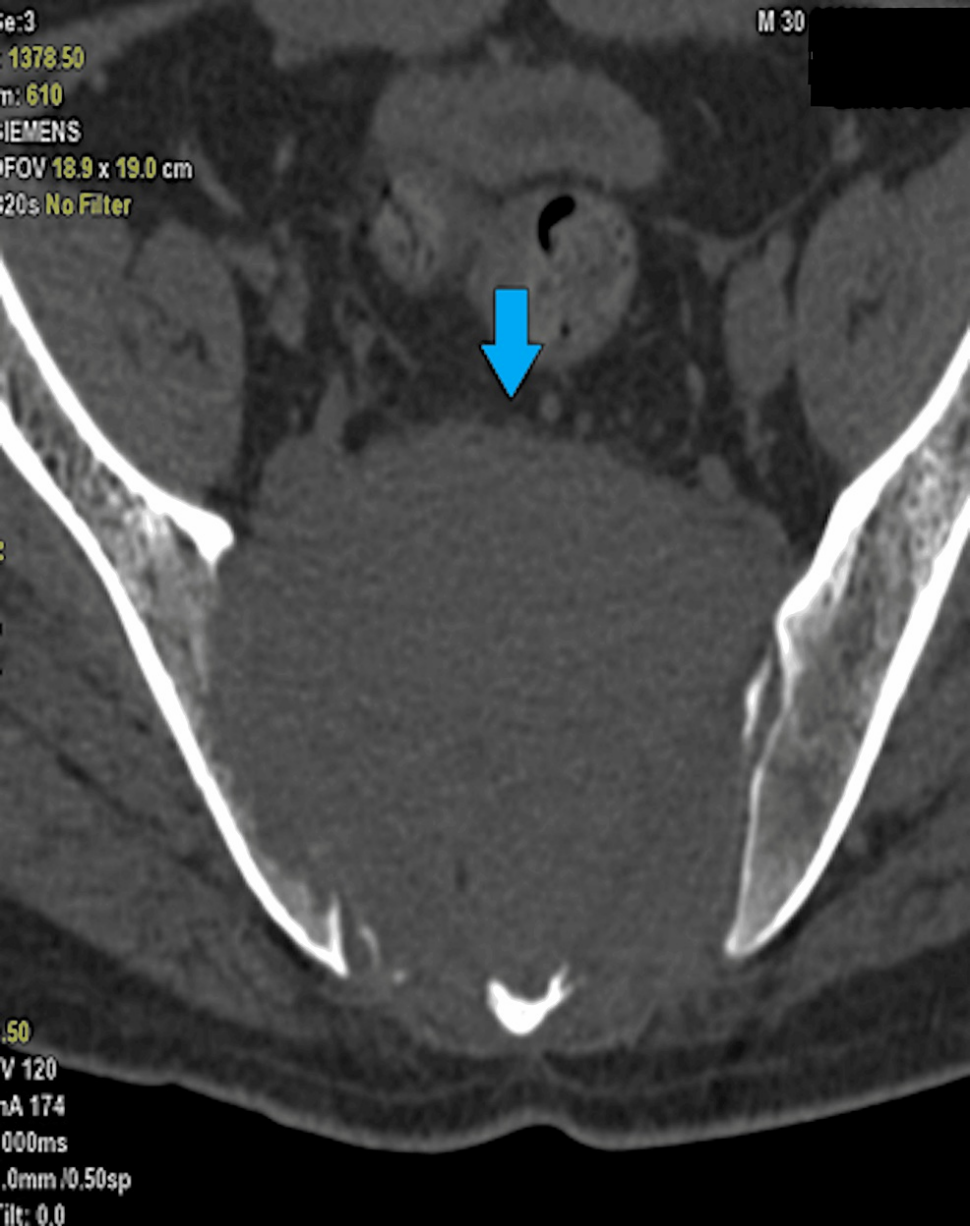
CT imaging in axial view showing a large giant cell tumour invading the sacrum and iliac bones (blue arrow). CT: computed tomography
